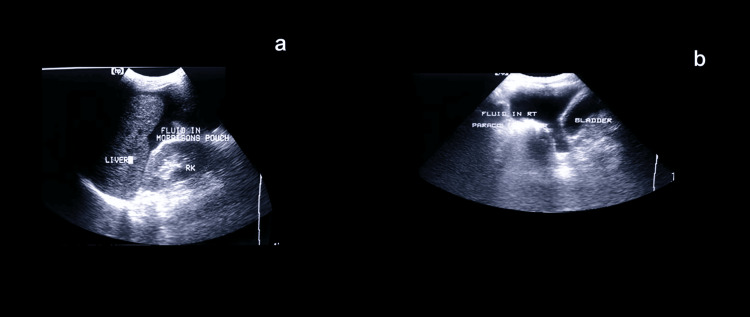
USG demonstrating hemoperitoneum (a) FAST image showing free fluid in Morrison’s pouch (hepatorenal recess) adjacent to the liver and right kidney. (b) Ultrasound image depicting free fluid in the right paracolic gutter surrounding the urinary bladder. FAST, Focused Assessment with Sonography for Trauma
